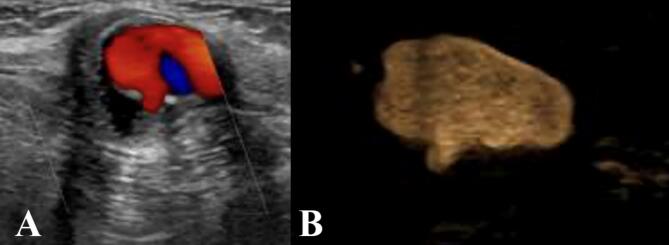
A and B: Respectively show US with Doppler-flow and B-mode images, revealing the defect in the prosthesis. B-flow mode focuses is designed to display the movement of blood as it flows through the vessel in a dynamic manner. It enhances the contrast between blood and surrounding tissue, enabling improved visualization of blood flow patterns and detection of abnormalities.
